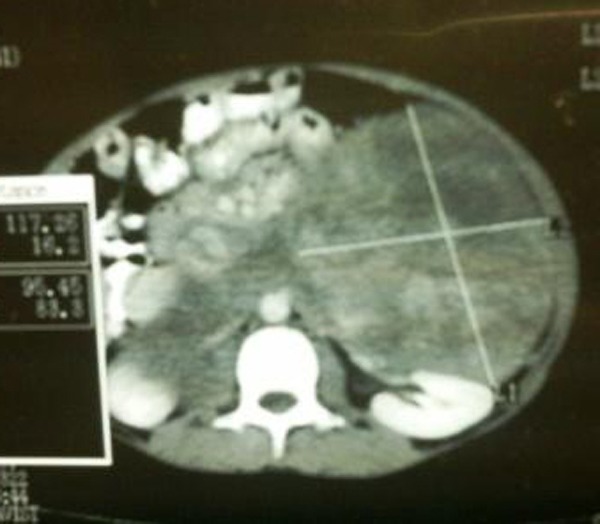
Figure 1: CT scan abdomen.
